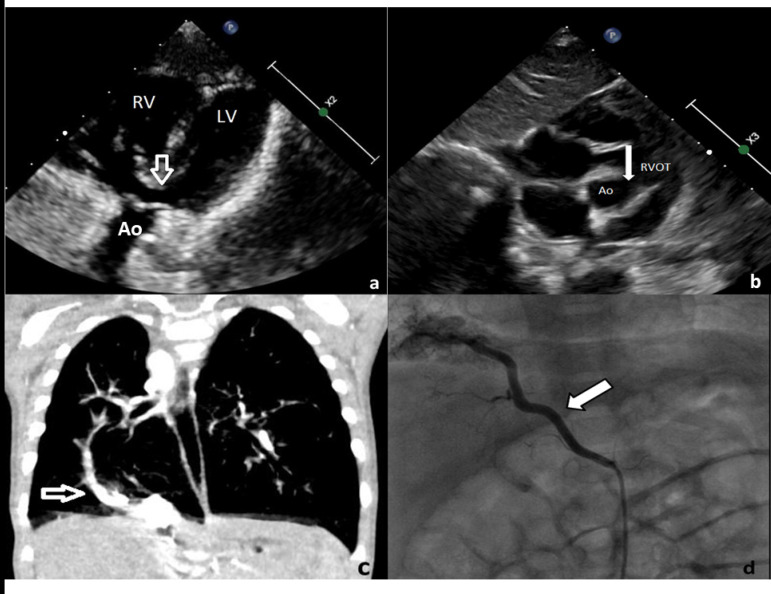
Echocardiographic 2D-modified subcostal view demonstrating (A) the subaortic VSD (white arrow), overriding aorta and right ventricular hyperthrophy, (B) modified parasternal short axis view displaying anterocephalic malalignment of the VSD (arrow) causing right ventricular outflow tract obstruction. (C) Computed tomography scan presenting abnormal right lower pulmonary vein (arrow) draining into the inferior vena cava, the pathognomonic “scimitar sign”. (D) Transcatheter angiography image of the sequestrating artery from the descending aorta. Ao=aorta; LV=left ventricle; RV=right ventricle; RVOT=right ventricular outflow tract
